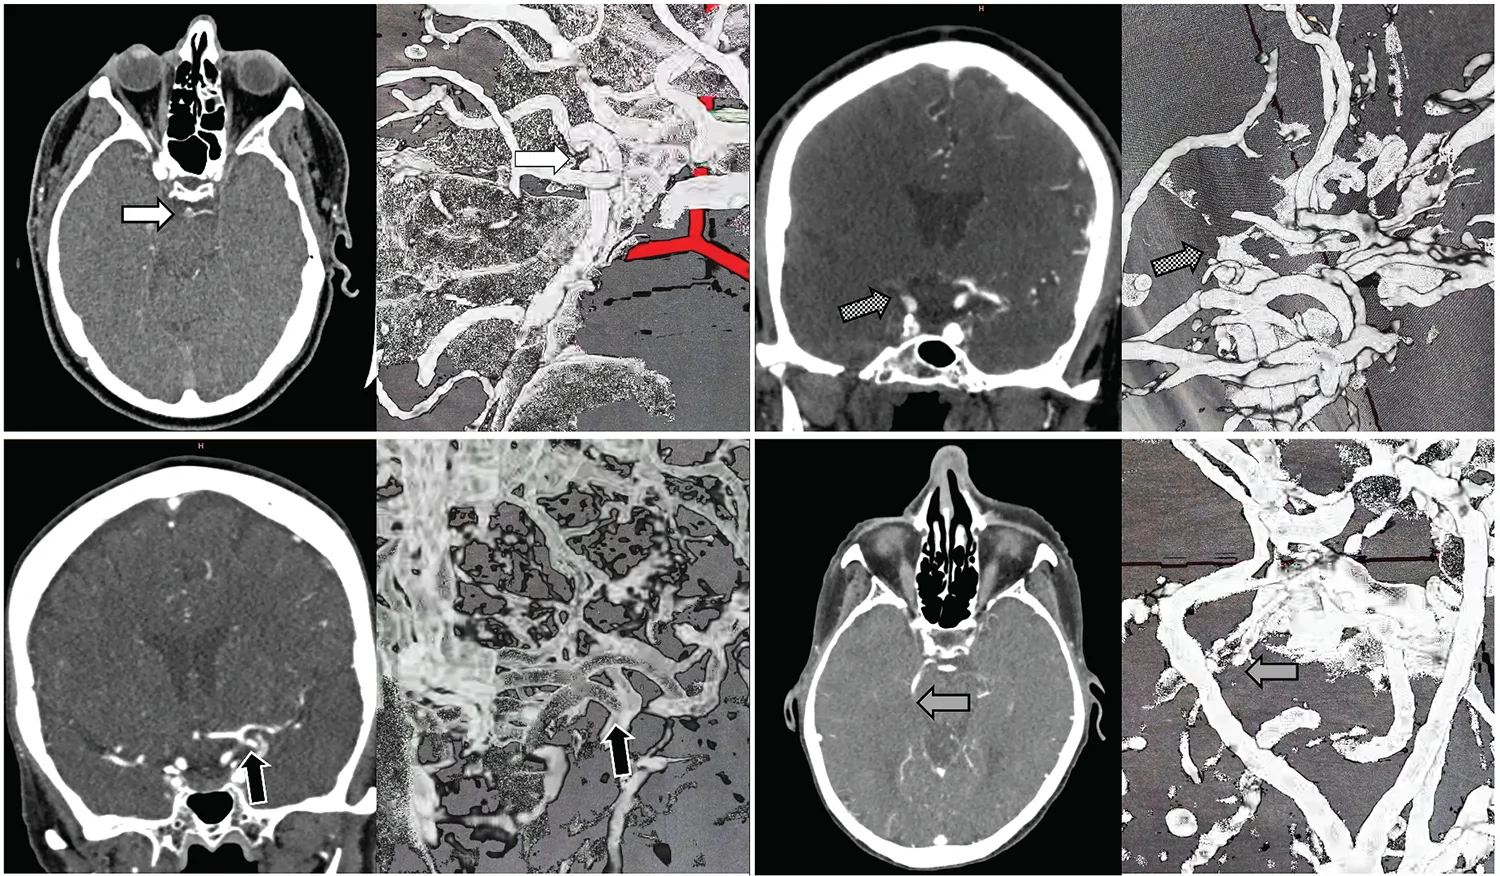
DICOM and Holo-Stroke-CTA views of challenging vessel occlusions. Figure 2 shows both DICOM and Holo-Stroke-CTA views of challenging vessel occlusions noted during this Holo-PACS experience. (Top-Left): White Arrow – Sub-occlusive thrombus in top of the Basilar artery. (Top-Right): Checkerboard Arrow – Right ICA occlusion. (Bottom-Left): Black Arrow – Duplicate Left MCA with inferior vessel occlusion. (Bottom-Right): Grey Arrow – Left PCA occlusion with overlying vein traversing in a similar path.
